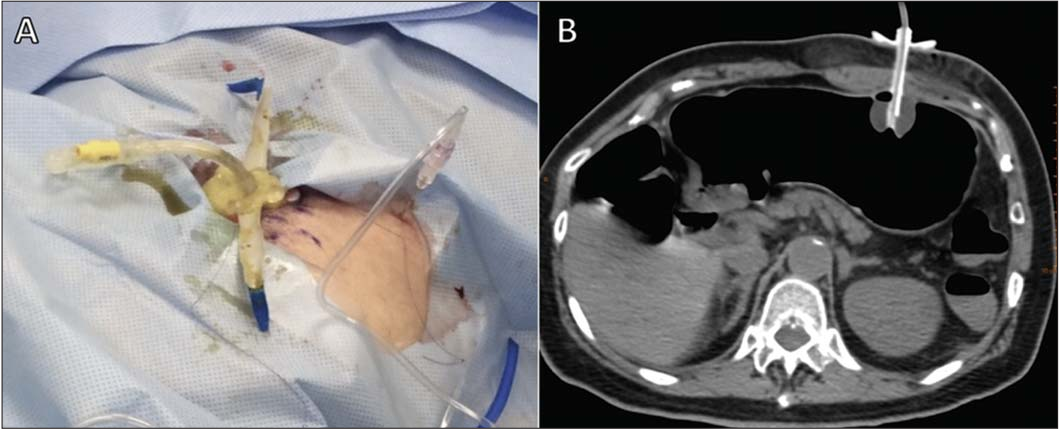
Introduction of the tube for the CT-guided percutaneous
